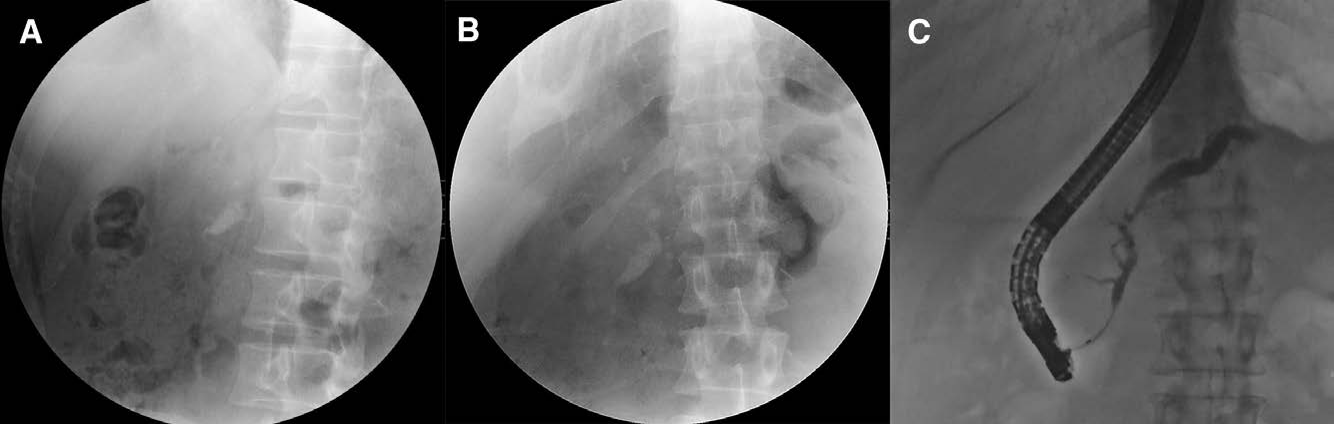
X-ray scan. (A) The X-ray image before second P-ESWL showed normal lower lobe of right lung. (B) The X-ray image after second P-ESWL showed patchy high-density shadows in the lower lobe of right lung (arrow). (C) The X-ray image before endoscopic retrograde cholangiopancreatography showed lung shadows were partially absorbed (arrow). P-ESWL = pancreatic extracorporeal shock wave lithotripsy.
